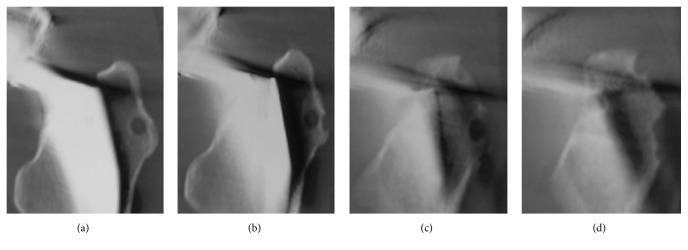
Coronal view of computed tomography showing osteolysis clearly ((a)–(d)).
